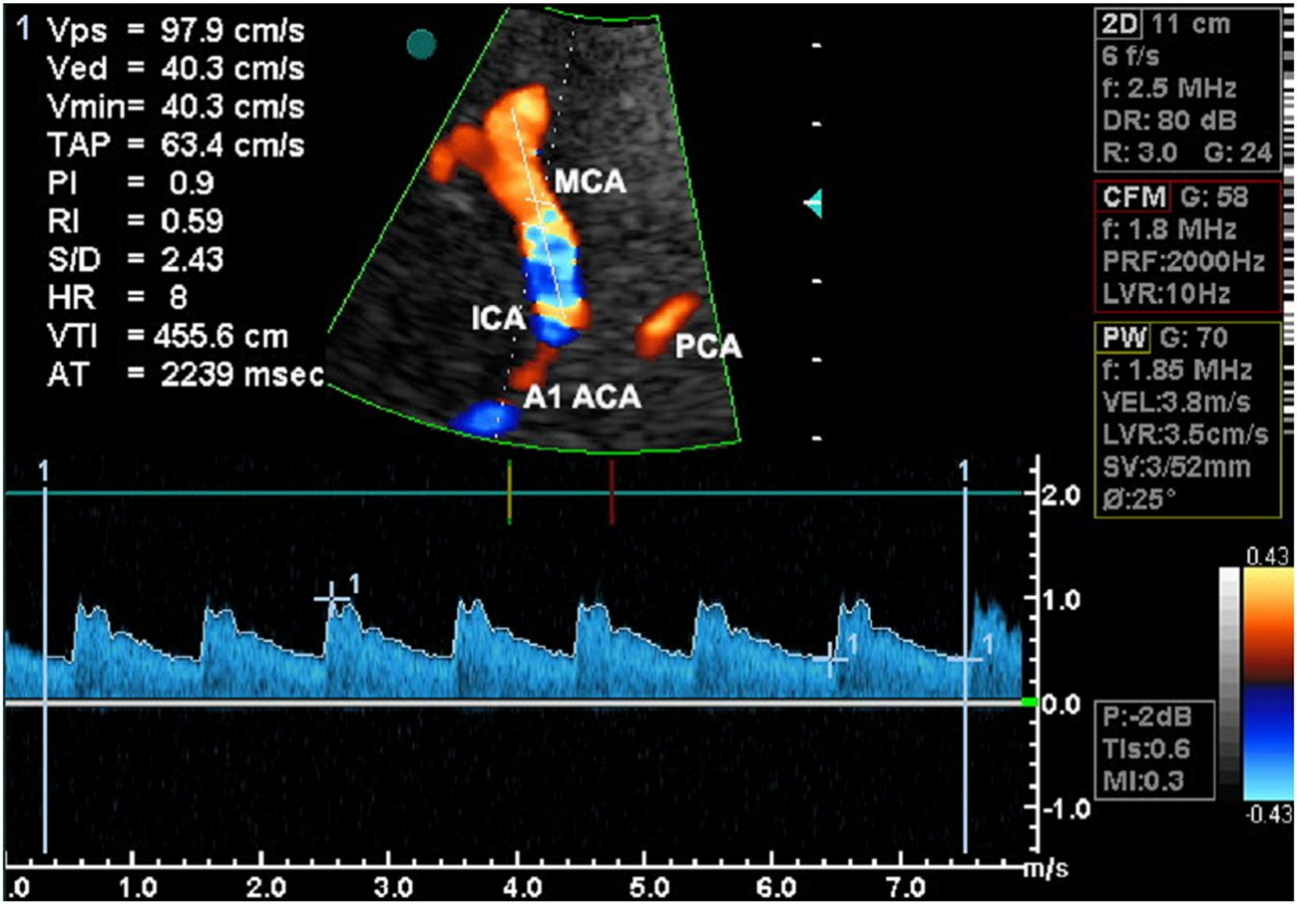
View of a color-coded image of the middle cerebral artery (MCA) with a corresponding Doppler spectral analysis performed using a transtemporal insonation. The sample volume was placed at a depth of 52 mm in the distal portion of the MCA. ICA, the internal carotid artery; PCA, the posterior cerebral artery; A1 ACA, A1 segment of the anterior cerebral artery.
